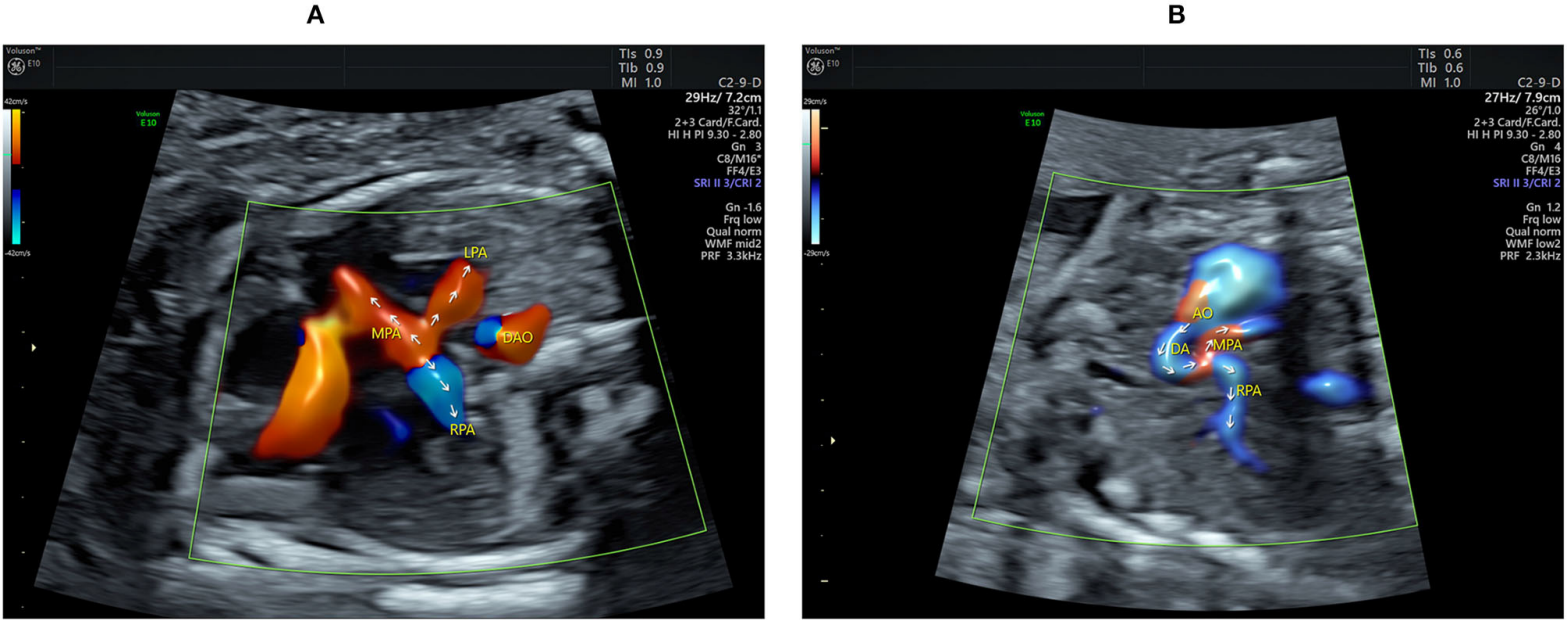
Echocardiograms showing the main pulmonary artery (MPA) with its branching and the aberrant ductus arteriosus (DA). (A) The Color flow mapping shows the antegrade flow in both the LPA and RPA together with the retrograde flow in the distal MPA (indicated by arrows). (B) The course and the direction of the blood flow (indicated by arrows) in the DA are clearly shown when using HDFI in a parasagittal view. AO, aorta; DA, ductus arteriosus; DAO, descending aorta; LPA, left pulmonary artery; MPA, main pulmonary artery; RPA, right pulmonary artery.
